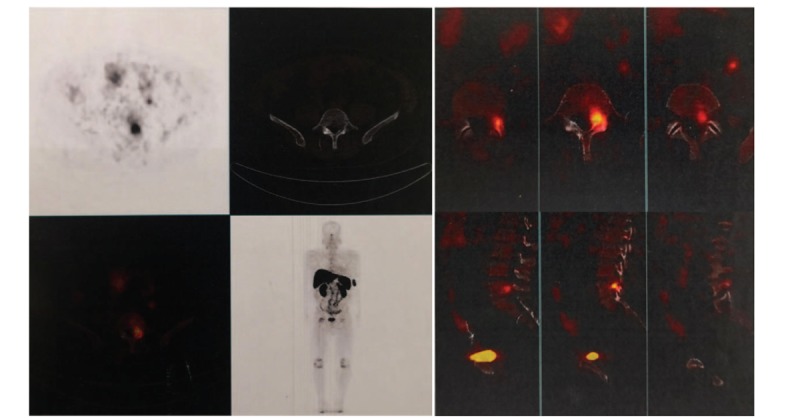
Positron emission tomography-computed tomography revealed the pathogenic tumor at L5.
